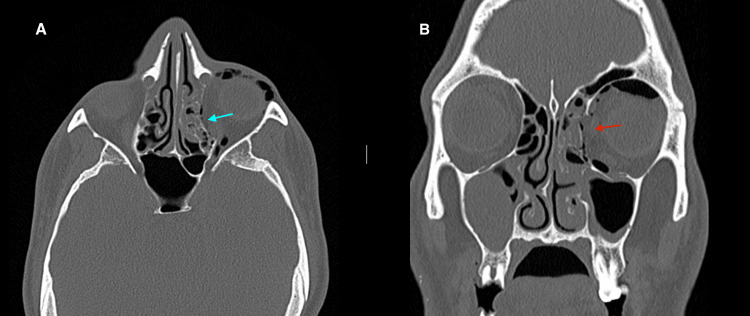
Axial and coronal CT of the facial bones Axial (A) and coronal (B) CT acquisitions with bone algorithms showing a large bone defect of the left lamina papyracea (red arrow) in the left medial wall fracture, with discontinuation of the lamina papyracea (blue arrow) and herniation of intraorbital fat tissue into the ethmoidal cells.
